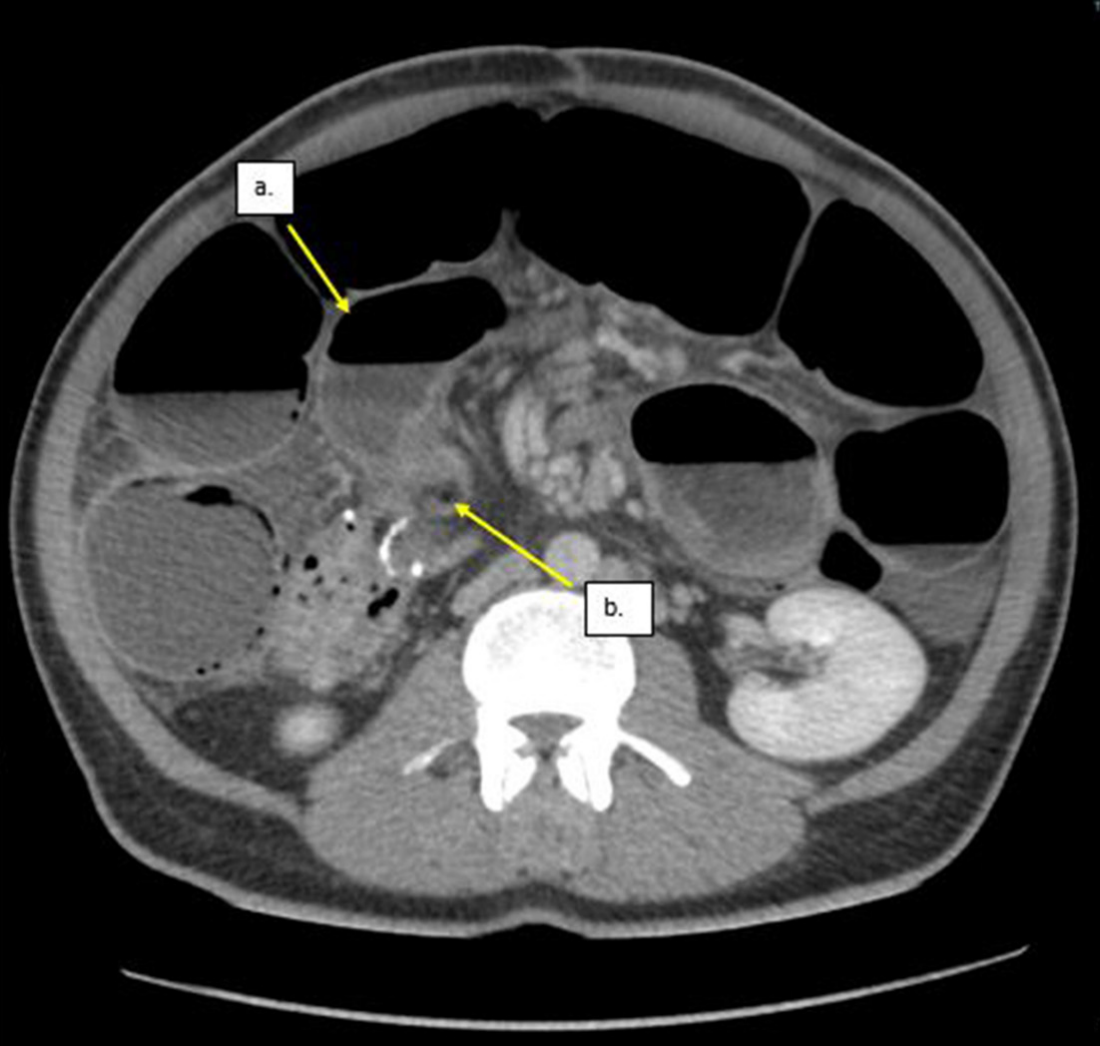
Axial CT demonstrating: (a) dilated fluid filled small bowel loops up stream to the acute transition point. b. Twisting of the small bowel around a fat density structure at the transition point, this fat density structure was demonstrated to represent a linear band on coronal images.
